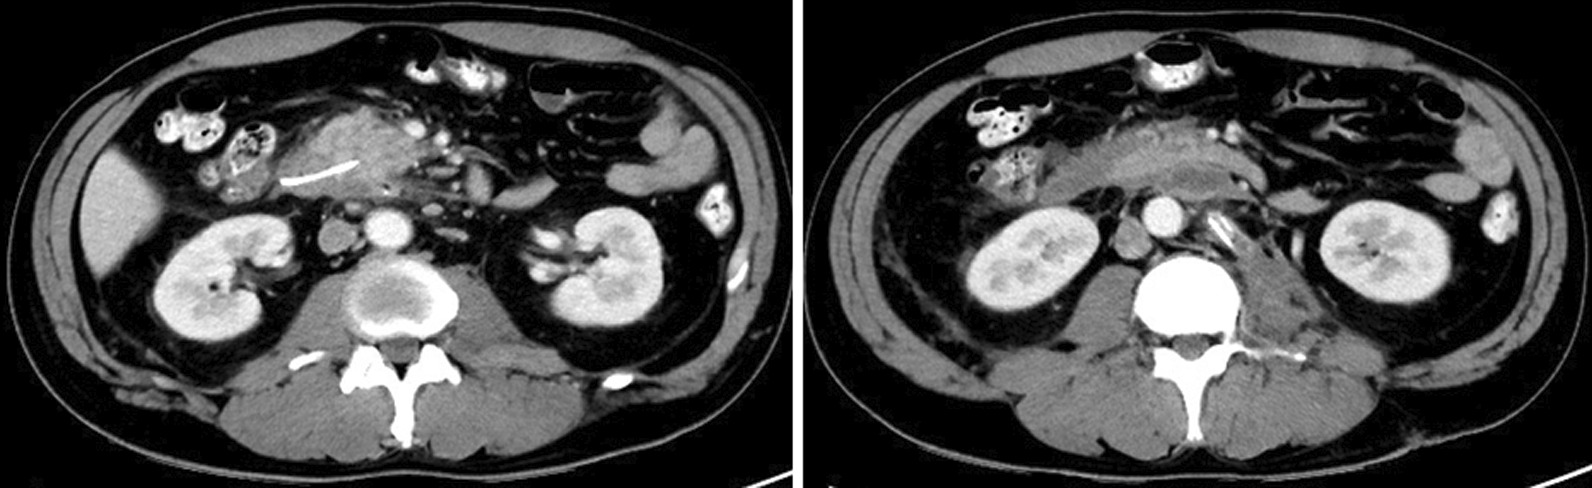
Computed tomographic image showing a marked reduction in the fluid collection after drainage.
